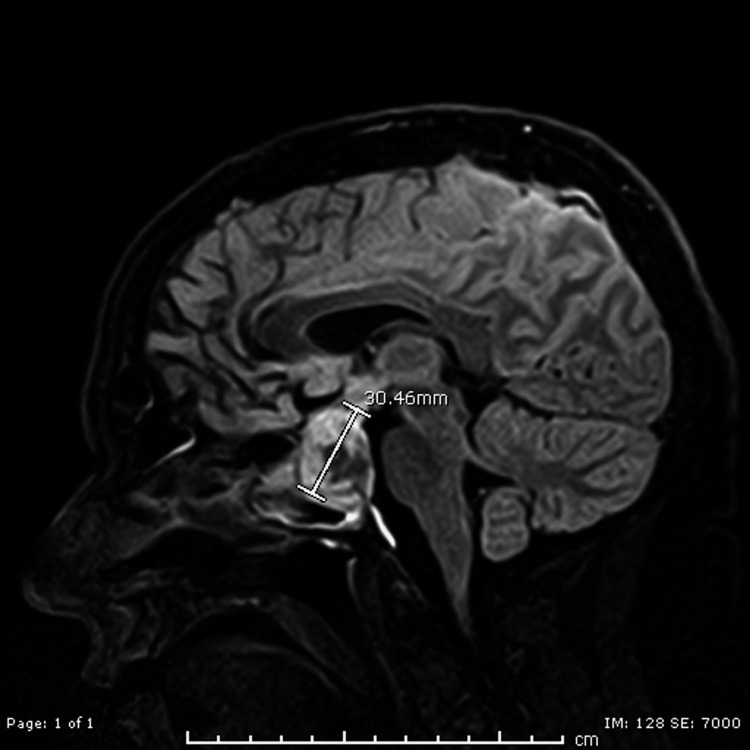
Sagittal cut of MRI brain DWI-FLAIR, which shows pituitary macroadenoma that measures 30.46 millimeters at its longest angle. MRI: magnetic resonance imaging; DWI-FLAIR: diffusion-weighted imaging and fluid-attenuated inversion recovery
